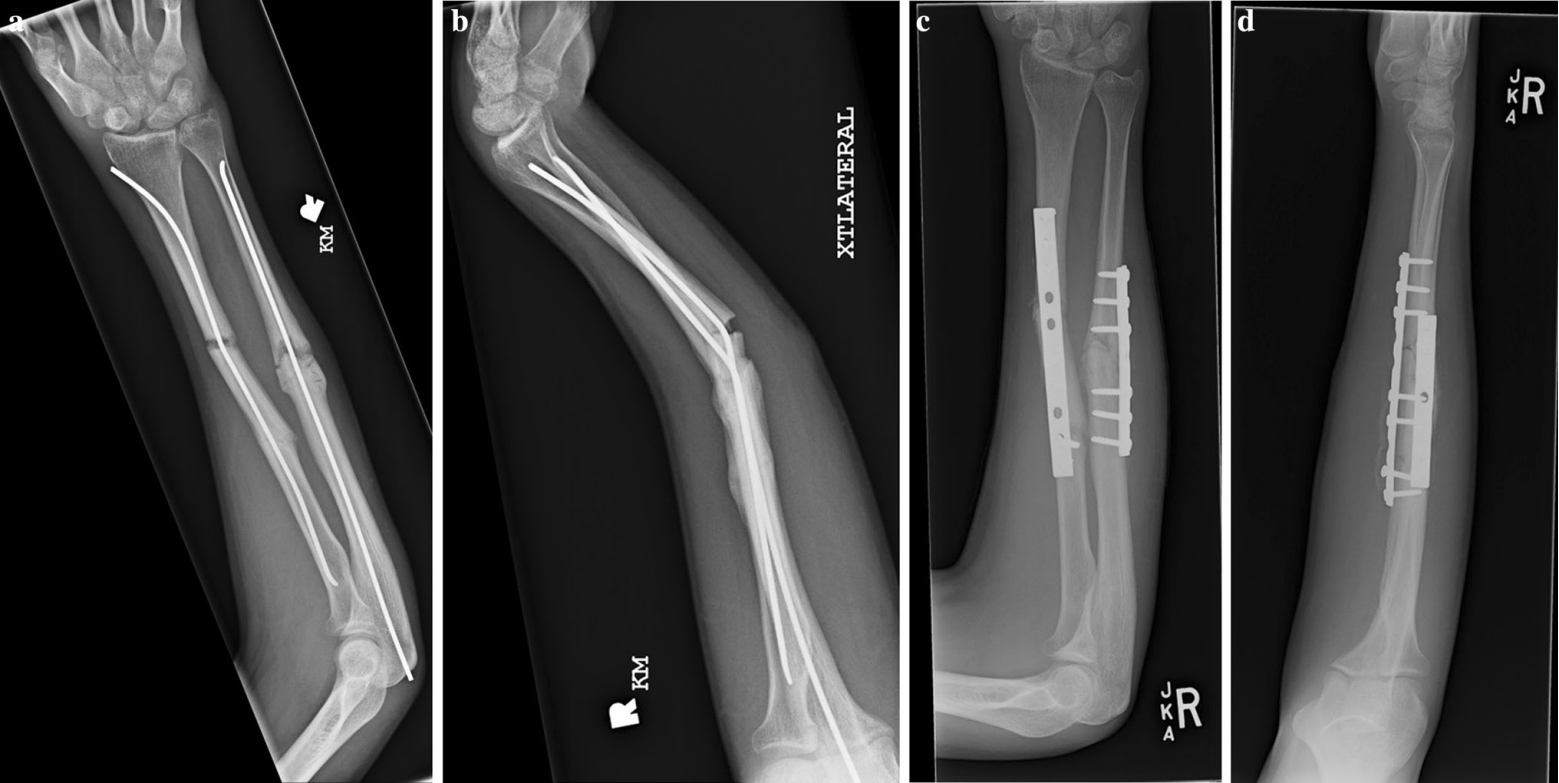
Anteroposterior (a) and lateral (b) radiographs of a 16 year old boy (Patient 2) who sustained a refracture while skateboarding. He was treated with removal of his implants and plating (c, d)
